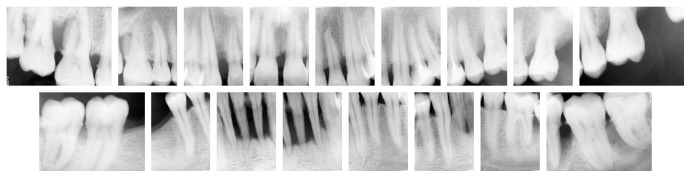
Full mouth periapical X-rays showing severe bone loss at lower anterior sextant and vertical bone loss at 13 and 37.
